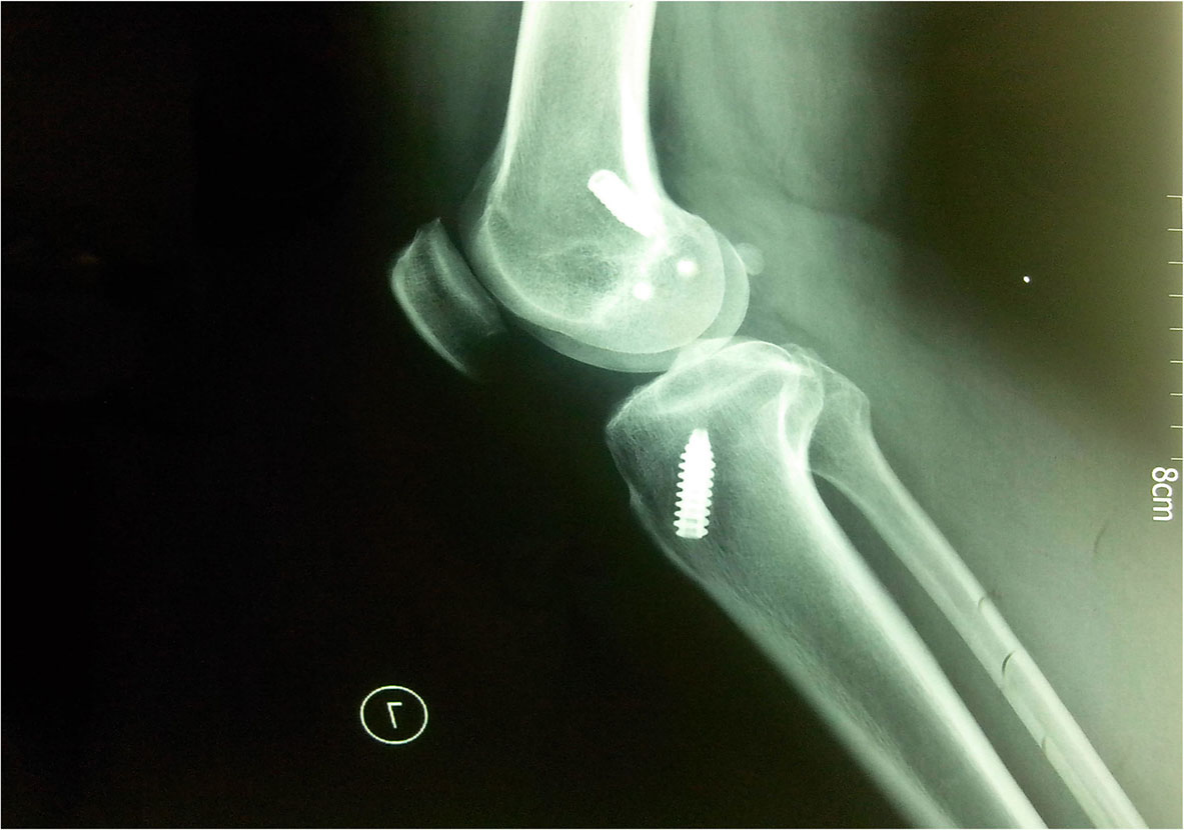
Preoperative X-rays demonstrated femoral and tibial tunnel placement in a lateral view
